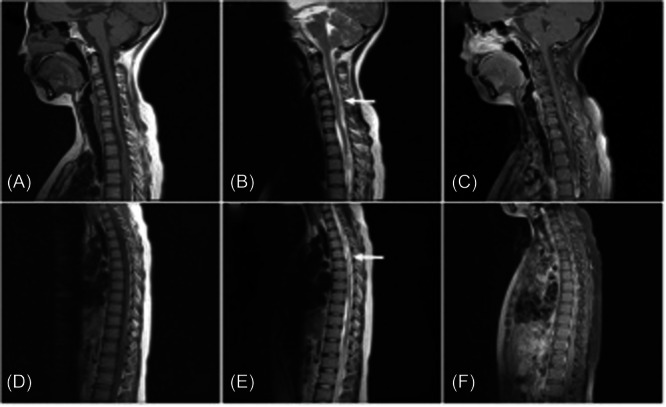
On September 18, an magnetic resonance imaging of the spinal cord displayed that multiple patchy mixed long T2 signal shadows were seen under the extramedullary epidural in the spinal canal from the lower edge of cervical vertebra 5 to thoracic vertebra 5, and the spinal cord was slightly deformed and displaced under compression (A–C). From the level of cervical vertebra 7 to thoracic vertebra 12, multiple patchy mixed long T2 signals were seen under intradural extramedullary, and patchy slightly long T2 signals were seen in the spinal cord of thoracic vertebra 3–5 (D–F). [Correction added on 20 May 2024, after first online publication: Figure 2 was revised in this version at the request of the authors.]
